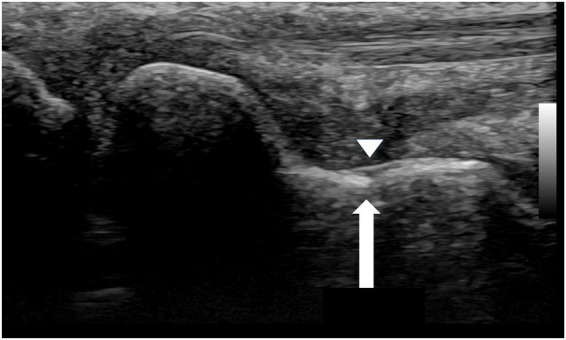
Follow-up ultrasound image of the waist region of the scaphoid (September 1, 2020). The white arrow indicates restoration of cortical continuity and formation of a bony bridge over the fracture site. The arrowhead demonstrates the bony callus formation.
